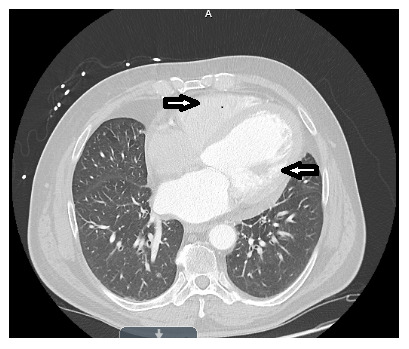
CT of chest with IV contrast showing left ventricular and right ventricular enlargement.
